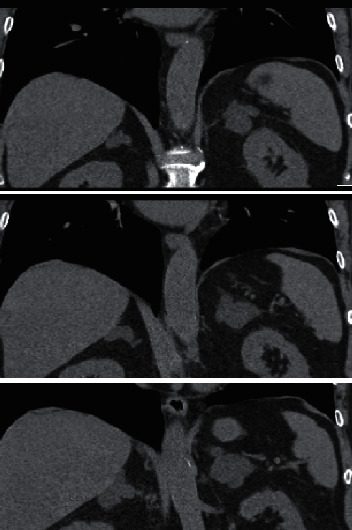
Adrenal CT scan of the index patient.
